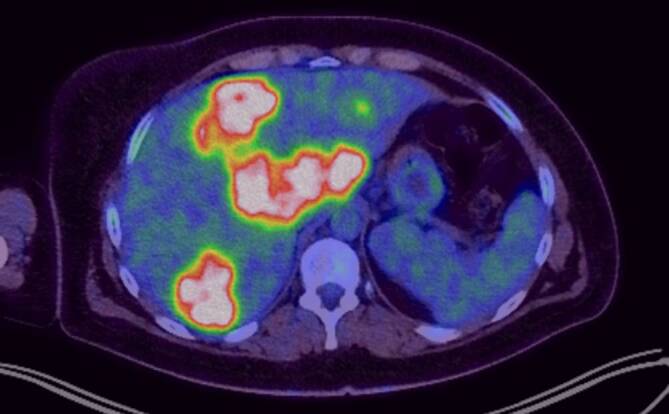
Onkologie-PET mit Low-dose-CT im Sagittalschnitt durch die Leber: deutliche Nuklidmehrspeicherung bei hepatischer Aussaat des KRK
